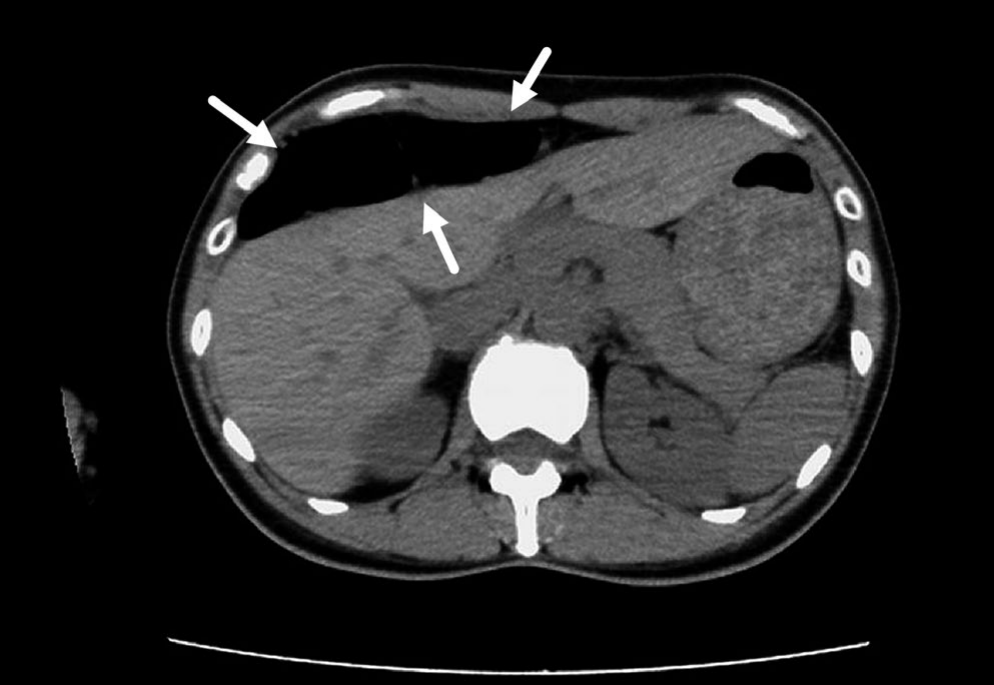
Abdominal computed tomography image without enhancement. The image shows the interposition of the colon between the anterior thoracic wall and the front edge of the liver (arrows), with no other abnormalities in the abdominal cavity
